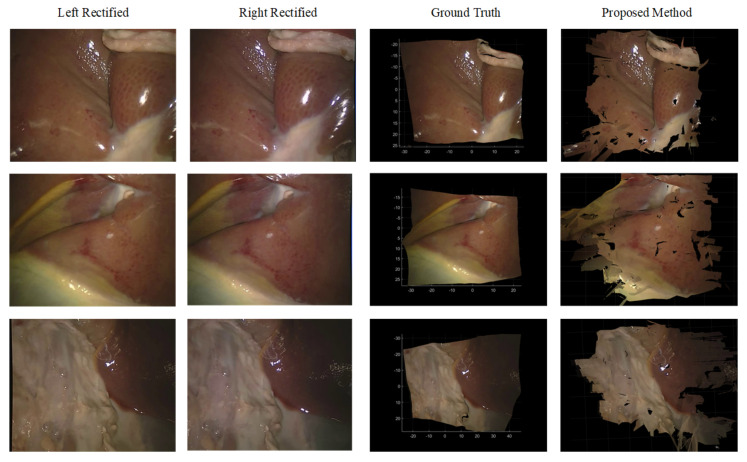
3D reconstruction results of SERV-CT datasets.
